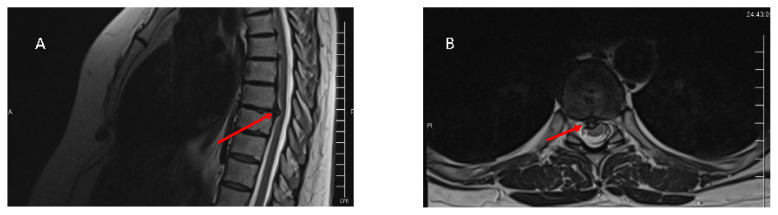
A 51-year-old female patient who presented with several months of back pain. Further investigation revealed a TDH at the level of T9–10. A. Coronal T2 image demonstrating TDH (red arrow) compressing the spinal cord at the same level. B. Axial T2 image demonstrating the same TDH (red arrow) compressing the spinal cord at the same level. Fortunately, there were no signs of myelopathy on the MRI scans or signs of radiculopathy at the patient’s presentation.
